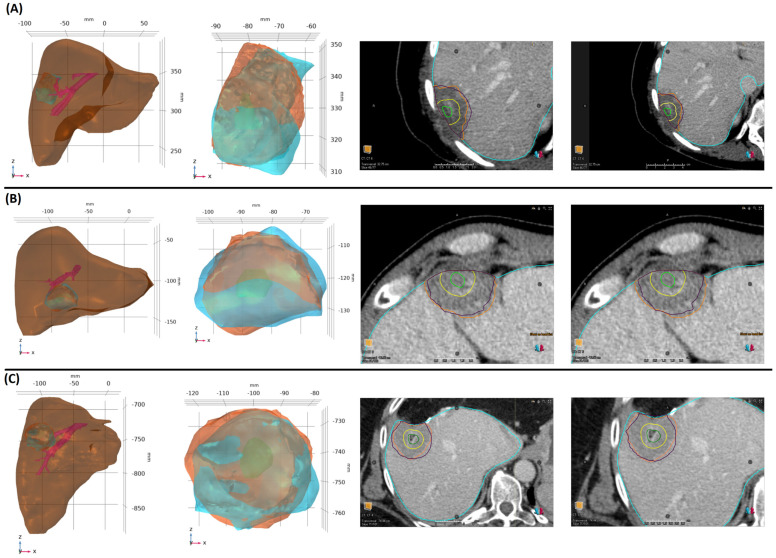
Patient-specific 3D models for patient 1 (A), patient 2 (B), and patient 3 (C). In each panel, the first image from the left shows the liver, tumor, and blood vessels, and the second image shows the clinical ablation zone (blue), computational ablation zone (orange), and tumor (green). The third and fourth images are post-ablation CT images showing the liver (blue), tumor (green), 5 mm MAM (yellow), clinical ablation zone (orange), and predicted ablation zone (purple) for ablation times of 10 min and 9.5 min, respectively.
